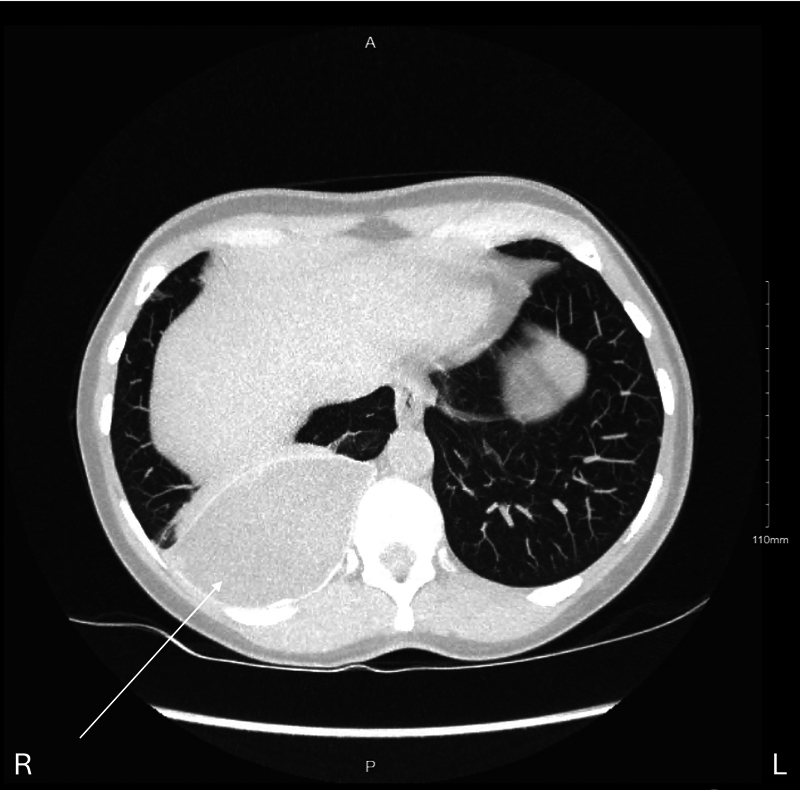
CT imaging demonstrating an intact breast prosthesis located in the right posterior basal pleural space (arrow).
